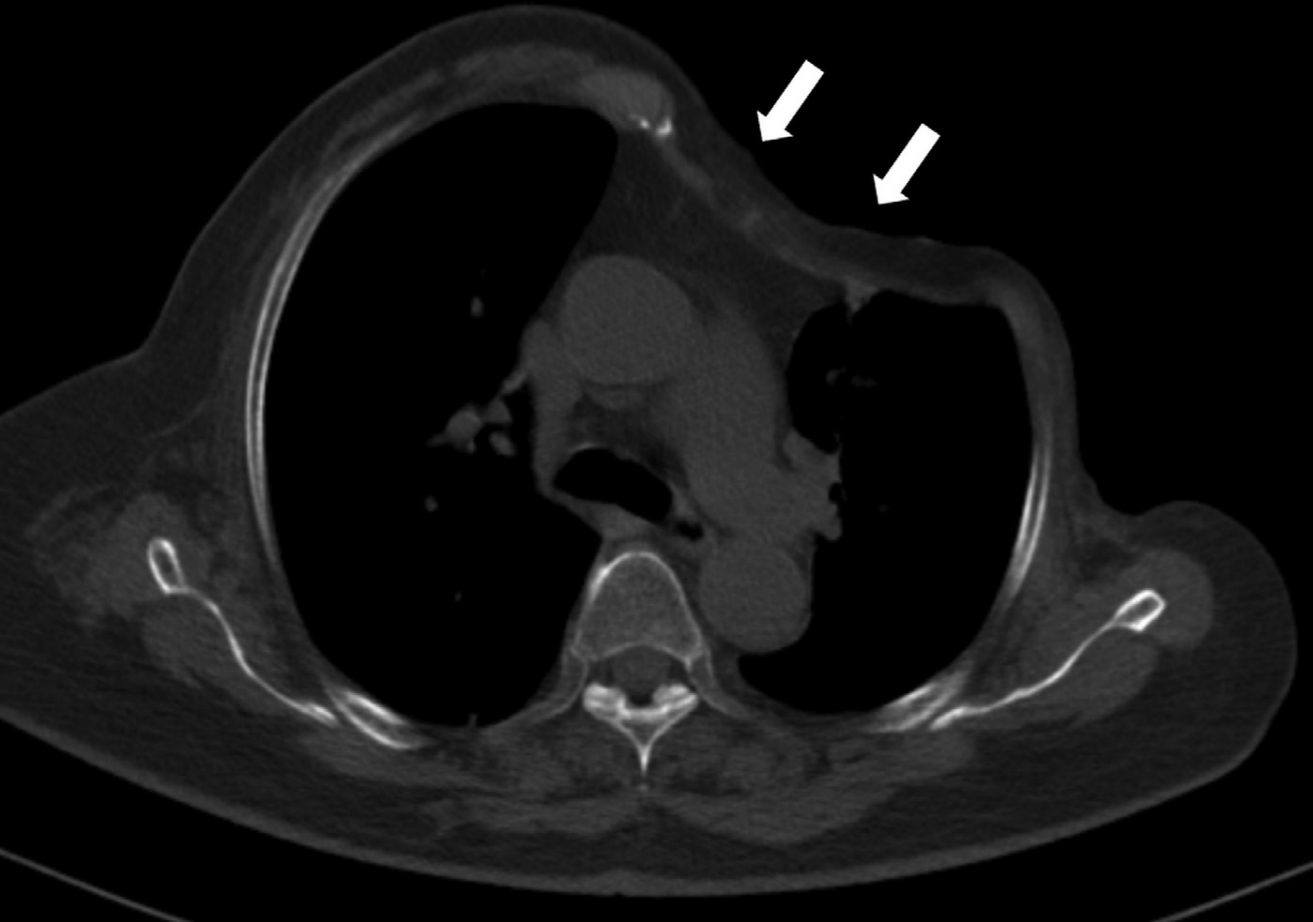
Radiological findings of osteoradionecrosis of the left anterior ribs in a 79-year-old woman. (D) Follow-up chest CT performed 7 months after reconstruction surgery revealed deformed left chest wall (arrows) without recurrence of ulceration or infection.
